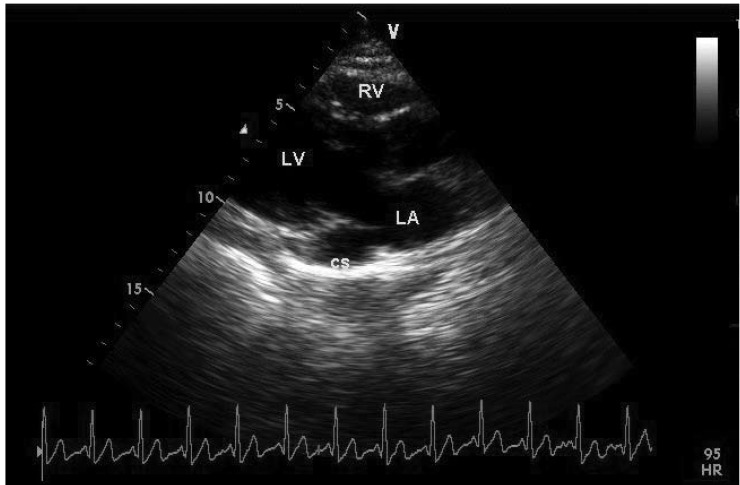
Dilated coronary sinus (CS) on transthoracic echocardiography, parasternal long-axis view (LA : left atrium, LV : left ventricle, RV : right ventricle).
